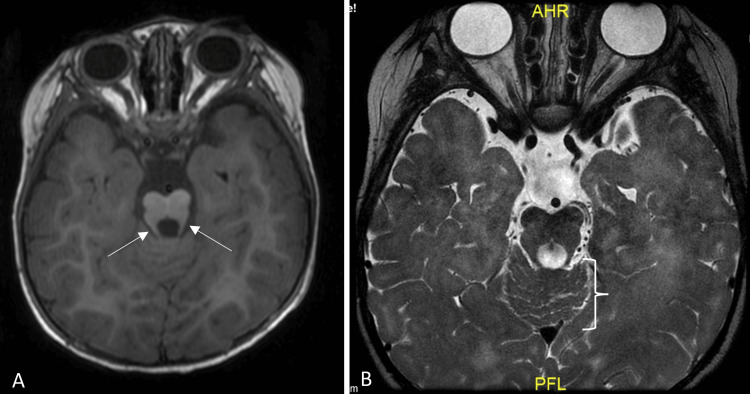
A: Mild lateral bulging and elongation of the superior cerebellar peduncles at the level of the pontomesencephalic junction, demonstrating a molar tooth configuration (arrows). B: Disorganization and anatomical distortion of the vermian lobules (bracket). A: Axial T1-weighted image of the brain at the level of the pons and vermis. B: Axial T2-weighted image of the brain at the level of the pons and vermis.
